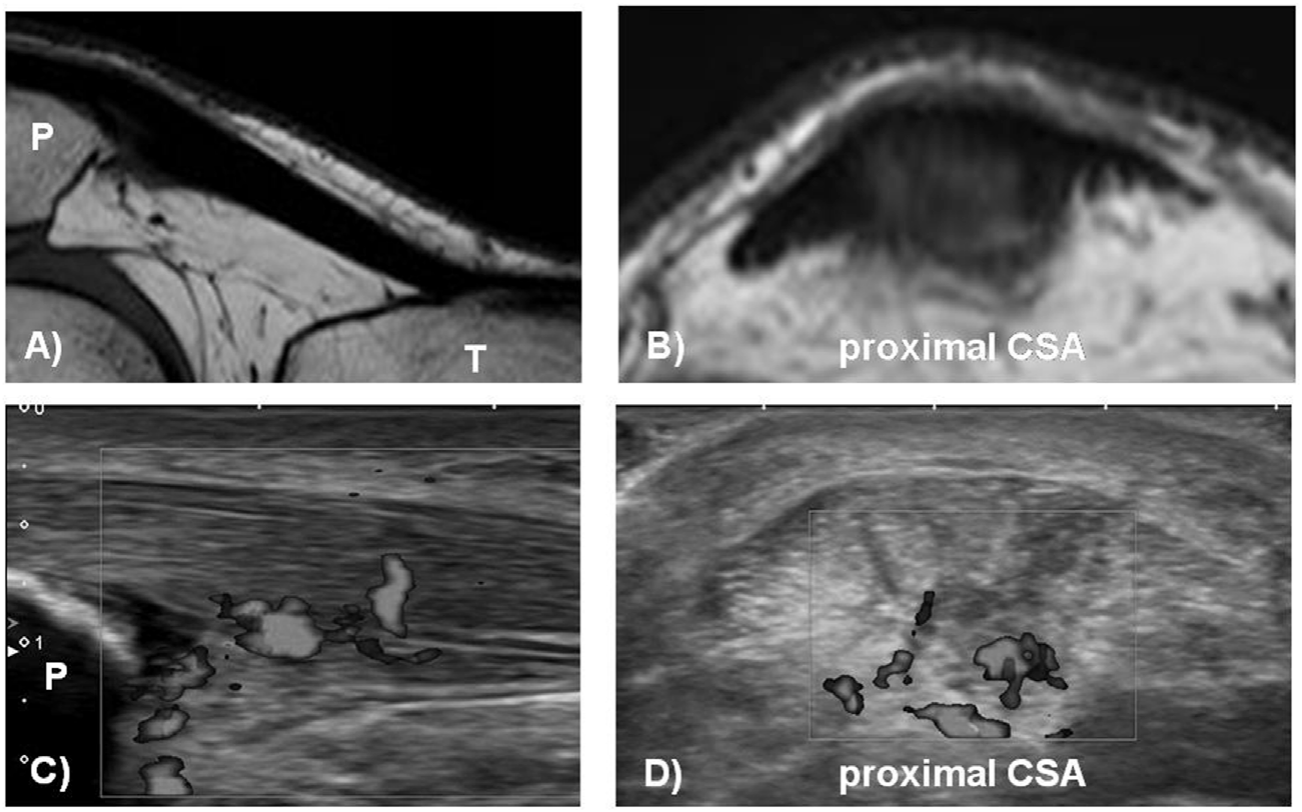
Magnetic resonance imaging [MRI, (A,B)] and ultrasound [US (C,D)] of tendinopathic patellar tendon in the sagittal and transversal plane showing tendon thickening [US (C) and MRI (A)], hypoechoic areas and neovascularisation (C,D) and increased signal intensity (B). CSA = cross-sectional area; P = patella; T = tibia.
